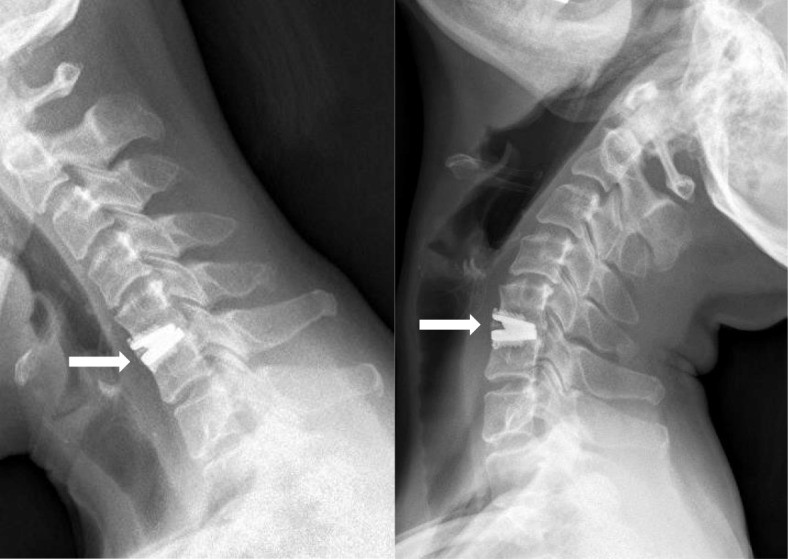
Lateral flexion and extension radiographs showing heterotopic ossification at C5/6 at 72 months after surgery
